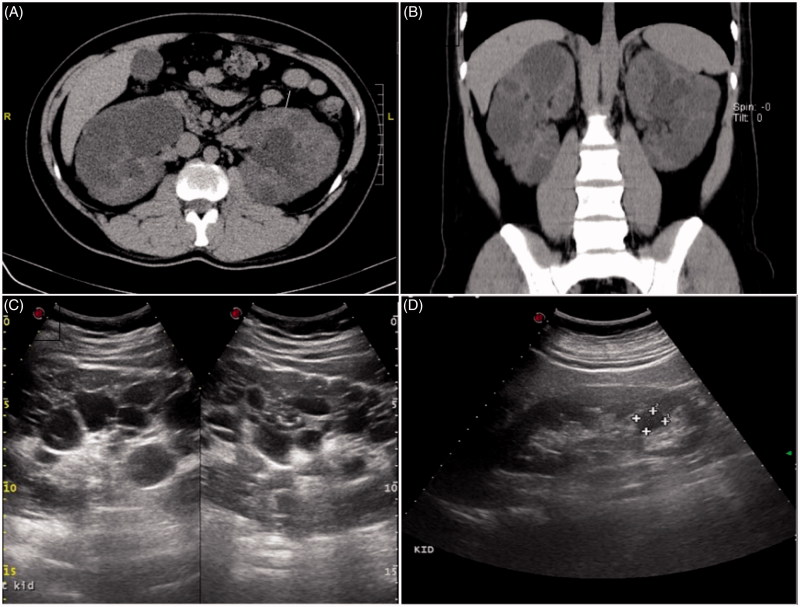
Representative computed tomography image of ADPKD patients. A and B are computed tomography imaging of (A) 38-year-old male patient P1 and (B) 49-year-old male patient P3. Kidney ultrasound images of P1 (C) and P3 (D).
